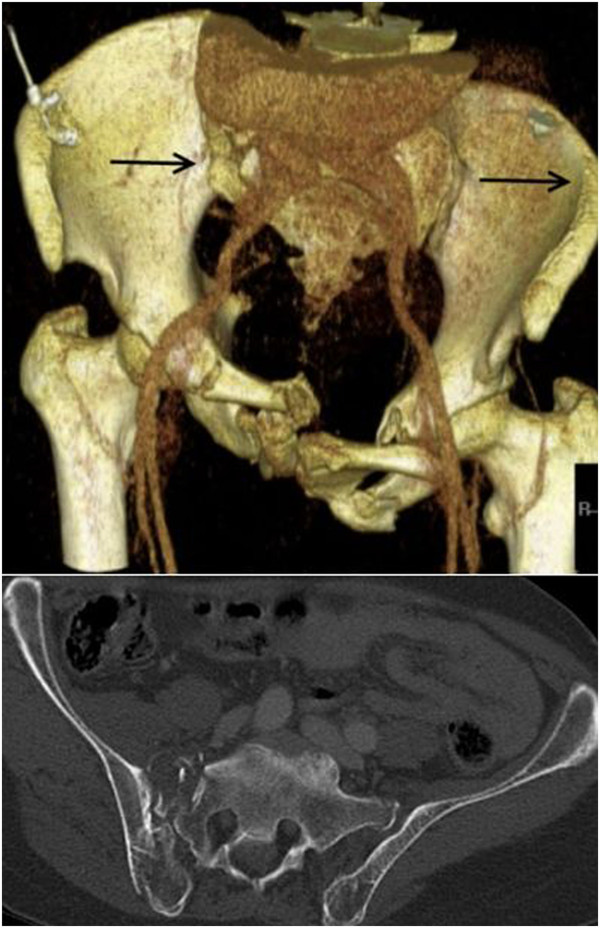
A 65 year-old patient was hit by a car as pedestrian. Computed tomography revealed a lateral compression type III pelvic ring fracture. The arrows mark the direction of force. In addition to restoration of pelvic ring instability, a median laparotomy was necessary duet to a concomitant laceration of the spleen Moore type II as well as an extra-peritoneal urinary bladder rupture.
